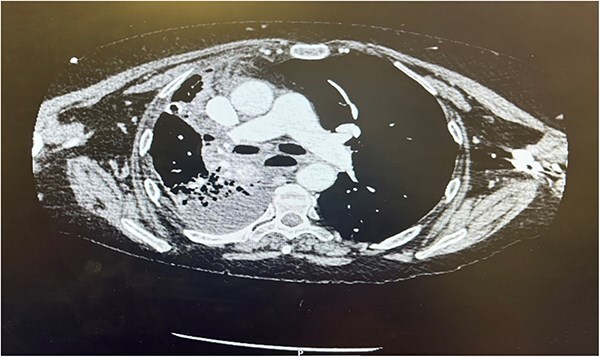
CT thorax Day 3 postoperatively. Right sided pleural effusion with overlying atelectasis and segmental collapse of the right middle and lower lobe.
